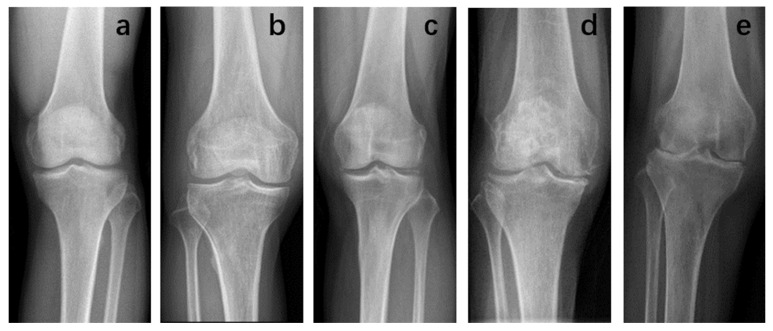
K-L classification. The patient was graded on an X-ray of the knee joint according to the method previously described. (a) Grade 0; (b) Grade I; (c) Grade II; (d) Grade III; (e) Grade IV.
